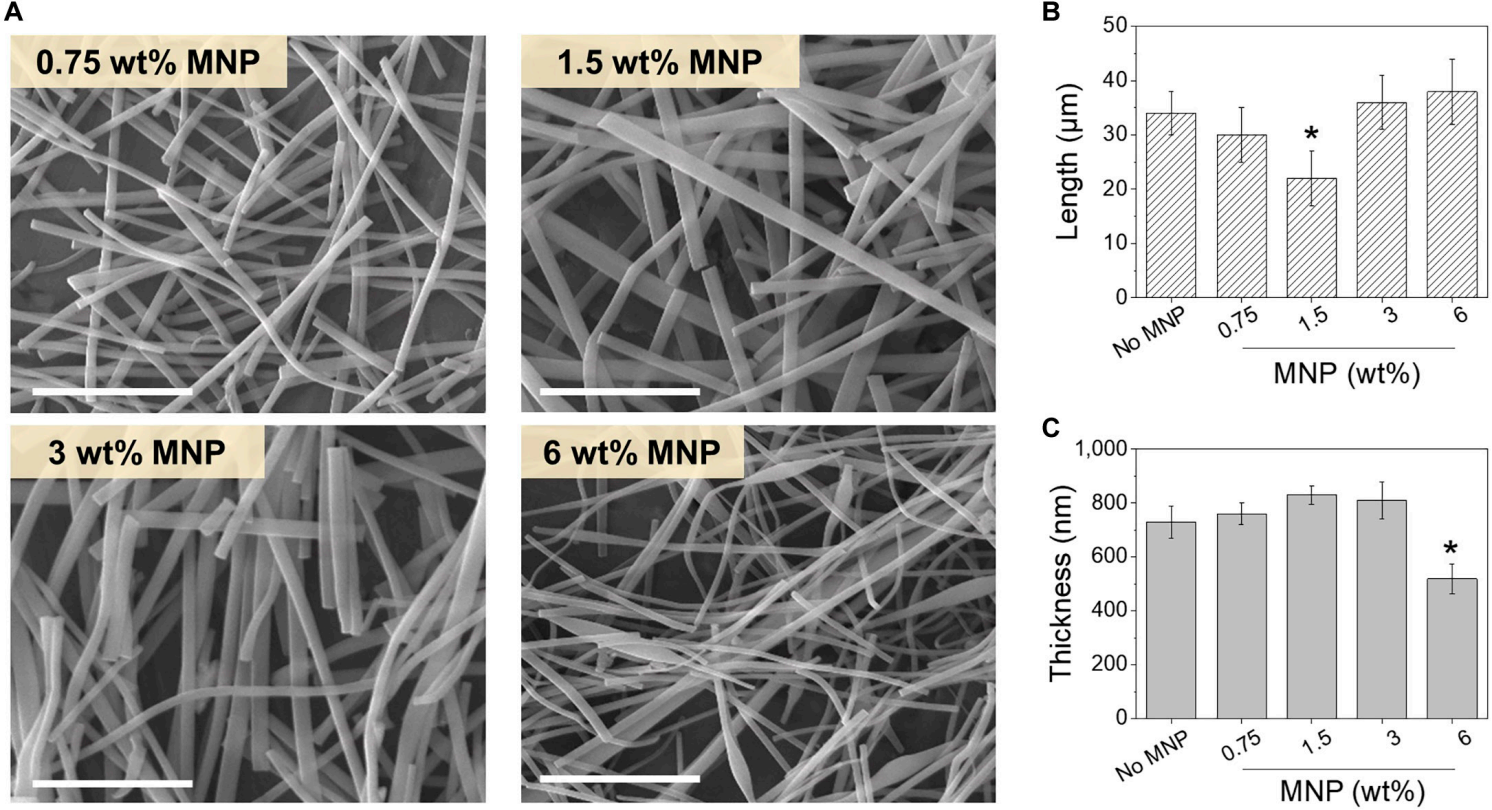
(A) SEM images of MNP-laden gelatin nanofibers cut by ultrasonication (scale bar: 10 μm). The average (B) length and (C) thickness of the short MNP-laden nanofibers (P* < 0.05 when compared with all other conditions, *n* = 10).
